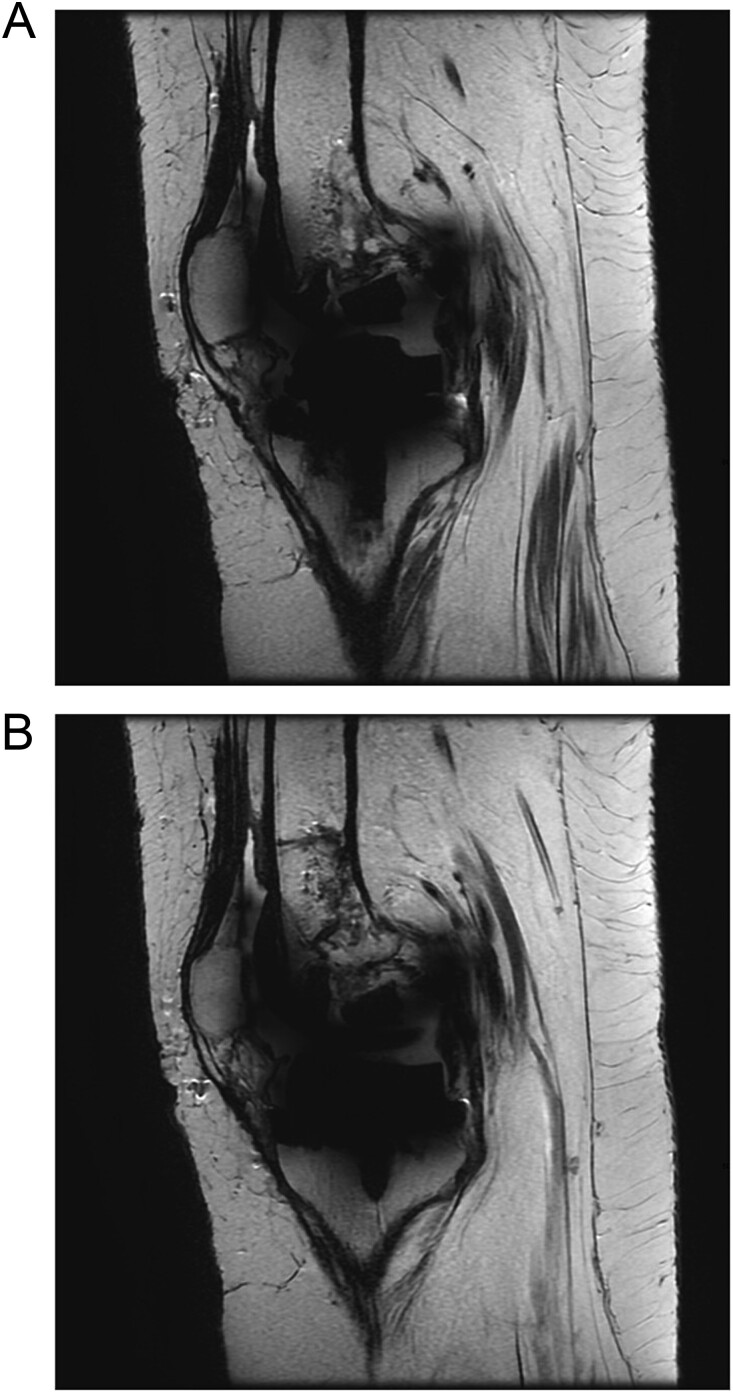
(A and B) Sagittal cuts from the MRI.
